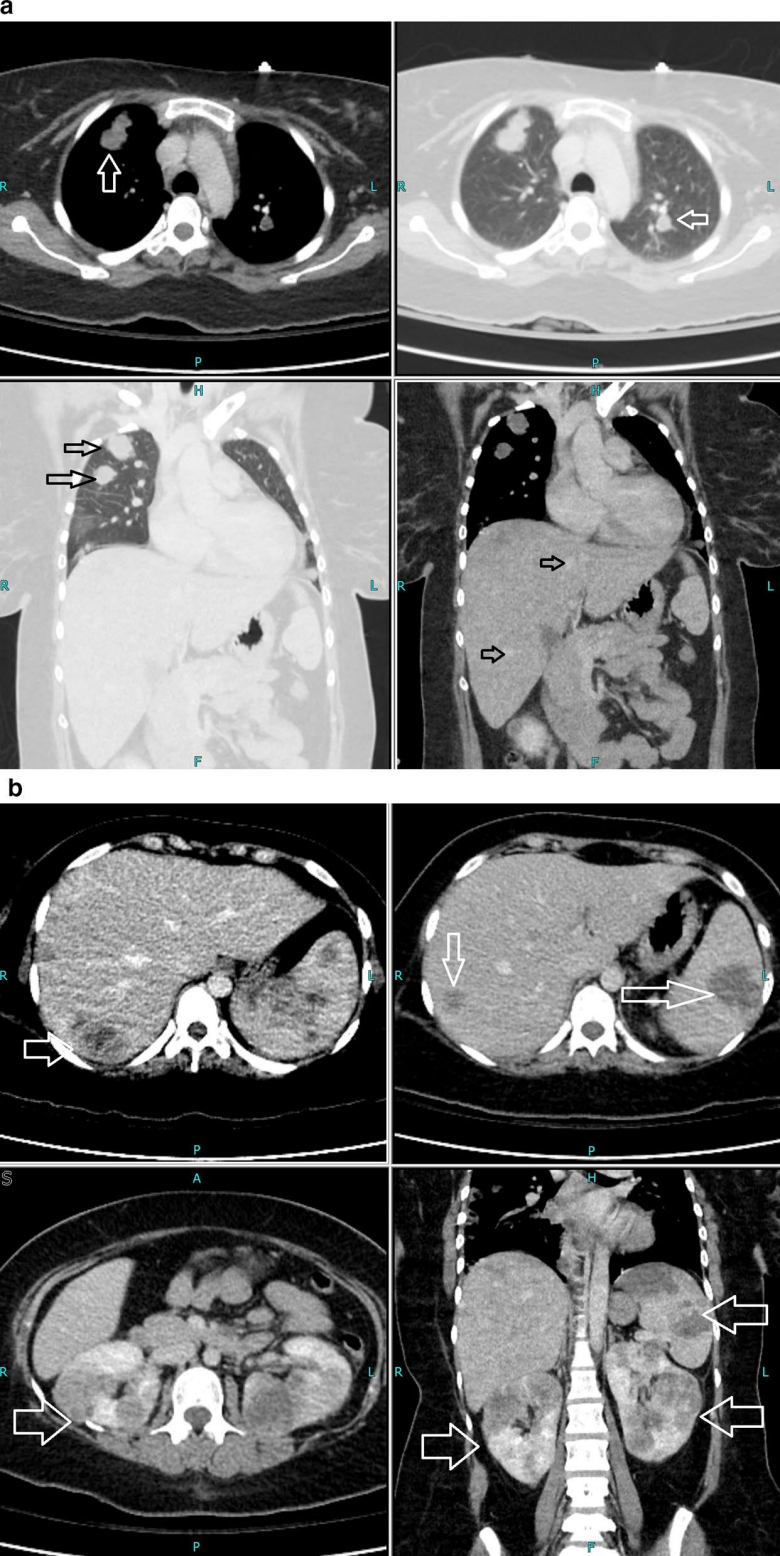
a Computed tomography of the chest showing neoplastic lesions in the lung (white and black arrows). b Computed tomography of the abdomen and pelvis (white and black arrows) showing multiple neoplastic lesions in liver, spleen, and kidneys
